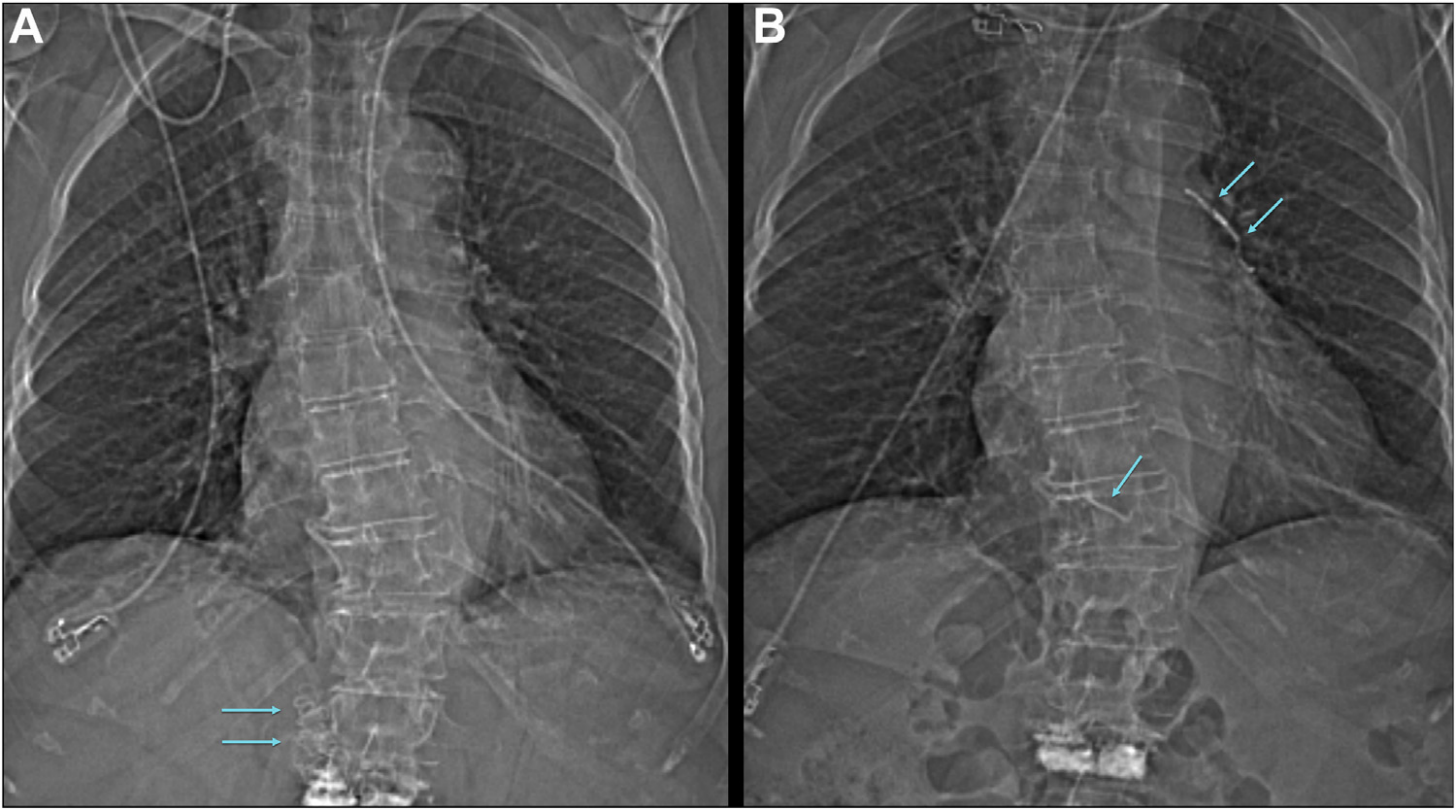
Anterior Posterior Topogram From Computed Tomography Scan (A) Cement casts in the inferior caval vein (arrows) before the ablation procedure, and (B) absence of cement casts in the inferior caval vein after the procedure with cement emboli (arrows) in the left pulmonary arteries and the right ventricle.
